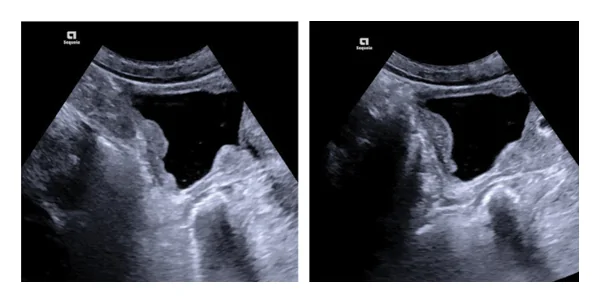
Ultrasound scan of the bladder at the time of diagnosis. Ultrasound showing thickened and irregular bladder walls and small capacity.
